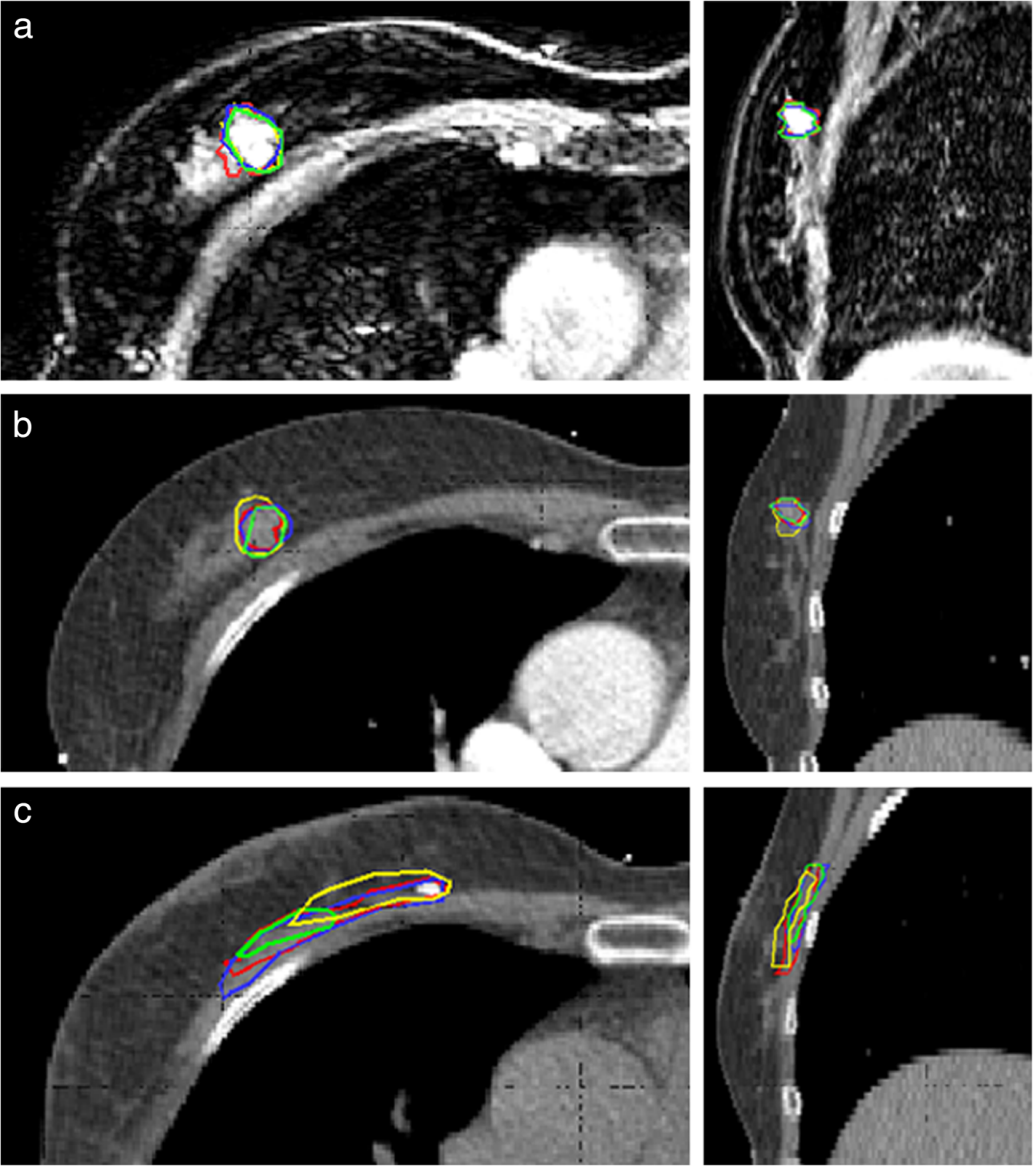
3D GTV delineations of 4 different observers in the transversal and sagittal plane in one patient on. (a) Preoperative CE-MRI (b) Preoperative CE-CT (c) Clinical postoperative CT.
